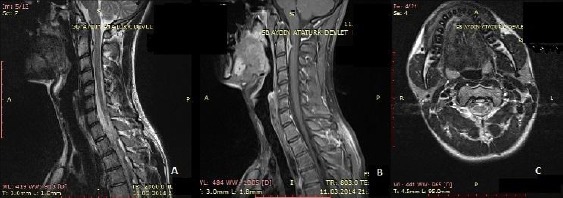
The control cervical MRI showing regression of meningioma in T2 weighted (A), T1 weighted sagittal (B) and axial (C) images*.
